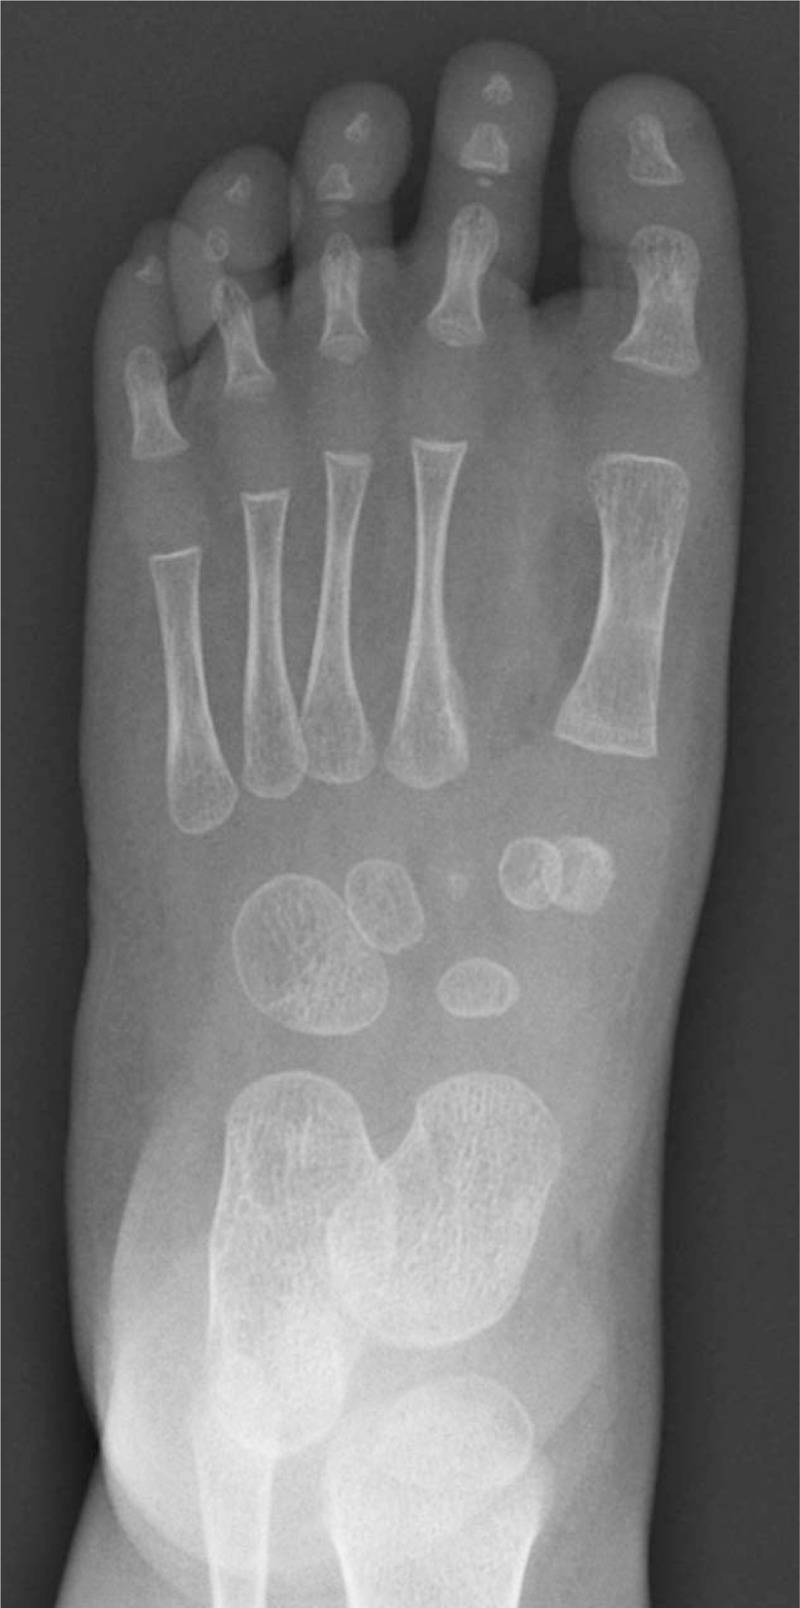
X-ray of final result (frontal plane).
